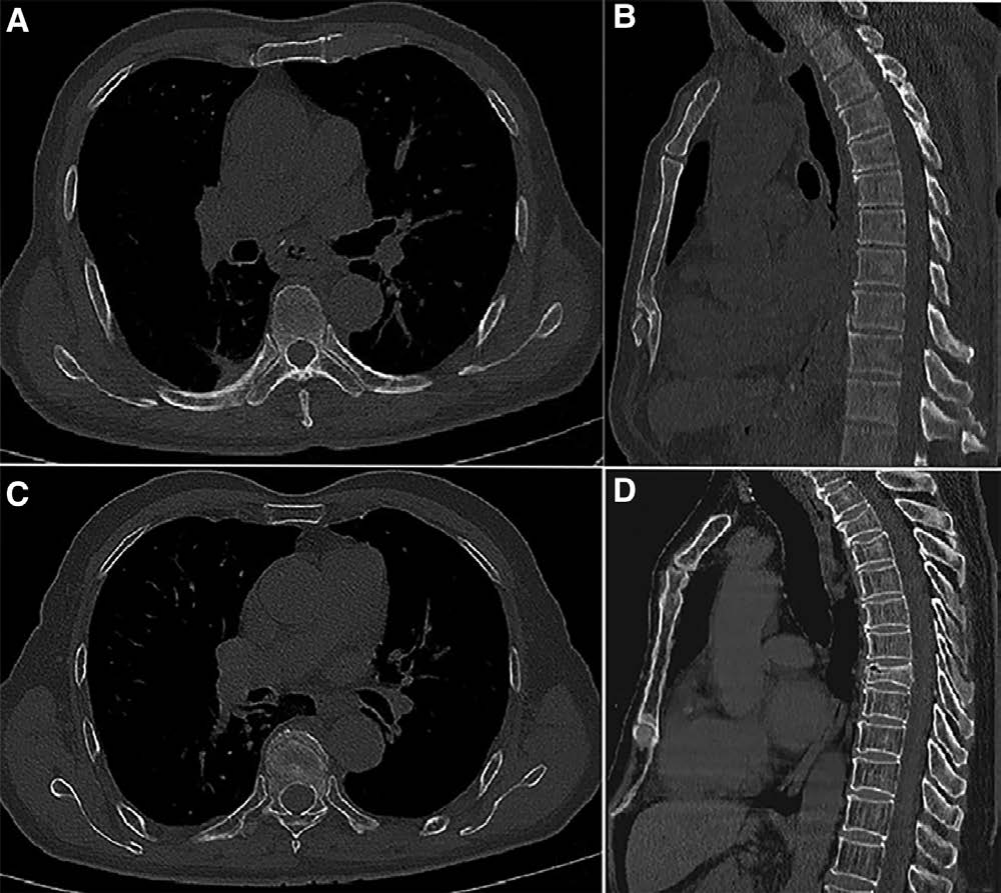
Axis and multiplanar reformation in sagittal views of computed tomography (CT) images before and after radiotherapy. (A and B) Initial CT images showing absence of thoracic vertebral fracture in Axial (A) and Sagittal planes (B) before radiotherapy. (C and D) Follow-up CT scanning showed T7 thoracic vertebral insufficiency fractures 23 mo after the radiotherapy.
